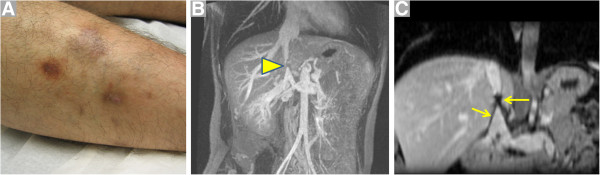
A 25 year-old Middle Eastern male with a 6 year history of recurrent deep venous thrombi despite therapeutic anticoagulation presented to a vascular medicine specialist. Physical examination demonstrated painful erythematous lesions over the lateral aspect of the calf (A, Courtesy Dr. Steven Dean) and oral ulcerations. B. Subsequent contrast-enhanced magnetic resonance venogram showed occlusion of a previously placed filter in the inferior vena cava (arrowhead). Extensive venous collaterals are evident. C. Coronal plane post-contrast volumetric interpolated breathhold T1-weighted image shows signal void delineating the IVC filter (arrows).
